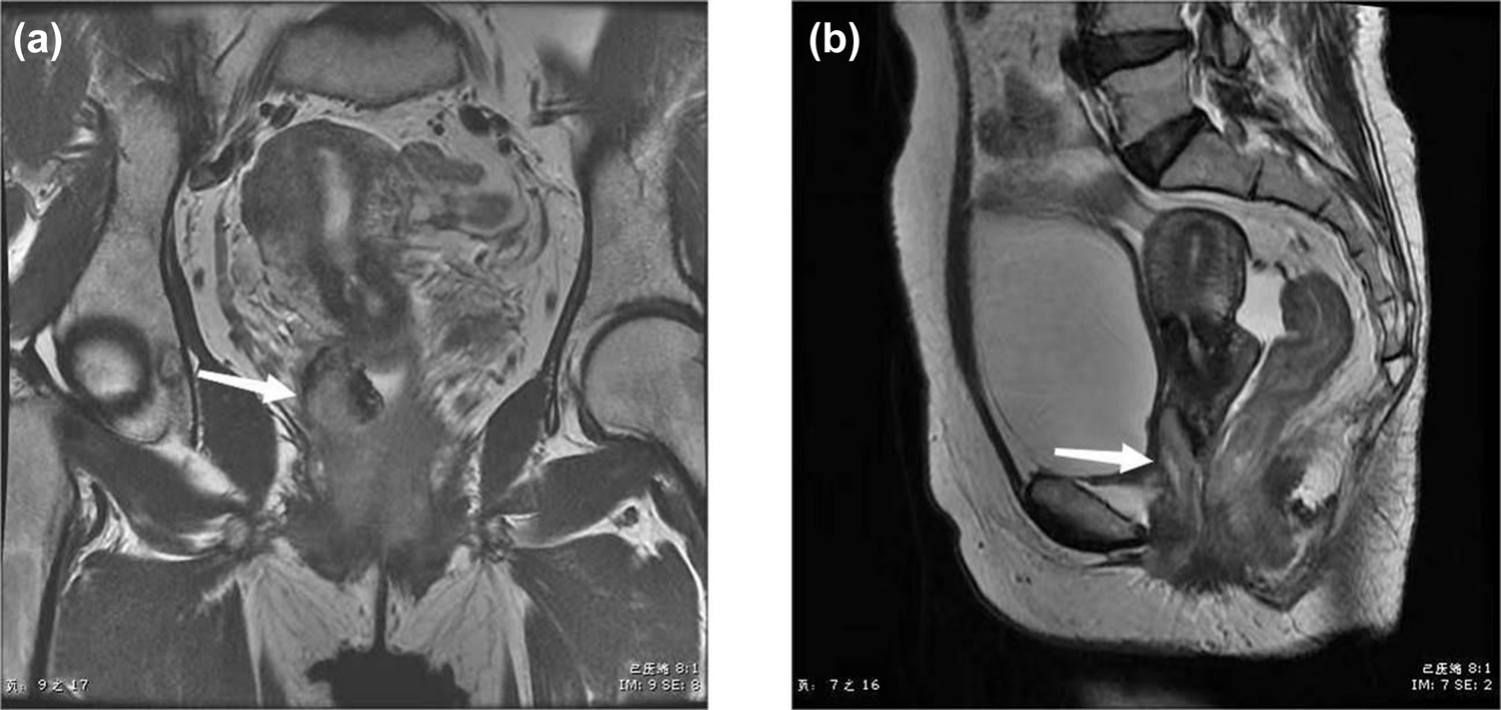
(a and b) T2-weighted coronal and sagittal magnetic resonance imaging (MRI) showed a mass in the right anterior wall of middle and upper segment of vagina (arrowhead).
